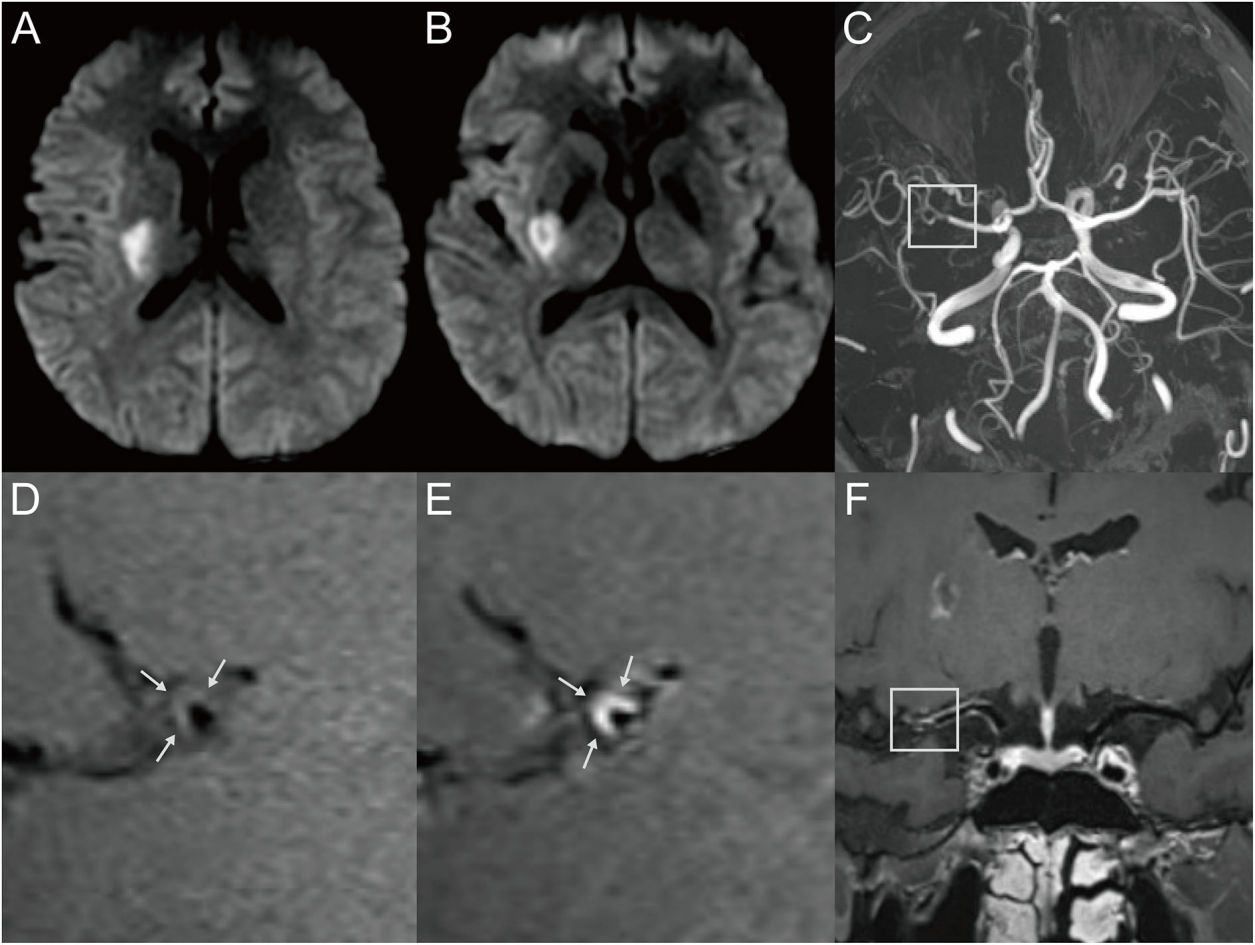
Representative MR images of a 34-year-old male patient with a single acute infarct lesion. The patient presented with left-side weakness for 2 days. The patient's risk factors included hypertension and hyperlipidemia. (A,B) The DWI images showed an infarct in the right basal ganglion region. (C) The 3D-TOF-MRA showed moderate stenosis of the right middle cerebral artery (MCA) (box). (D) The T1-weighted HRMRI demonstrated an eccentric atherosclerotic plaque with iso-signal intensity at the corresponding location (arrow), indicating probable parent artery atherosclerosis occluding a penetrating artery. (E) Postcontrast T1-weighted HRMRI showed strong enhancement of the plaque (arrow). (F) The postcontrast T1-weighted image by curved planar reformation showed the location of the plaque, which involved the superior wall where the perforator originated (box).
